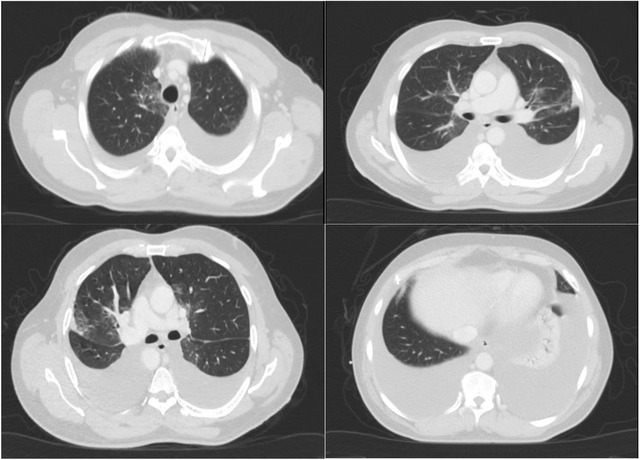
CT thorax showing significant bilateral pleural effusion with associated collapse/ consolidation of the bases of both lungs and areas of ground‐glass appearances and incomplete consolidations
